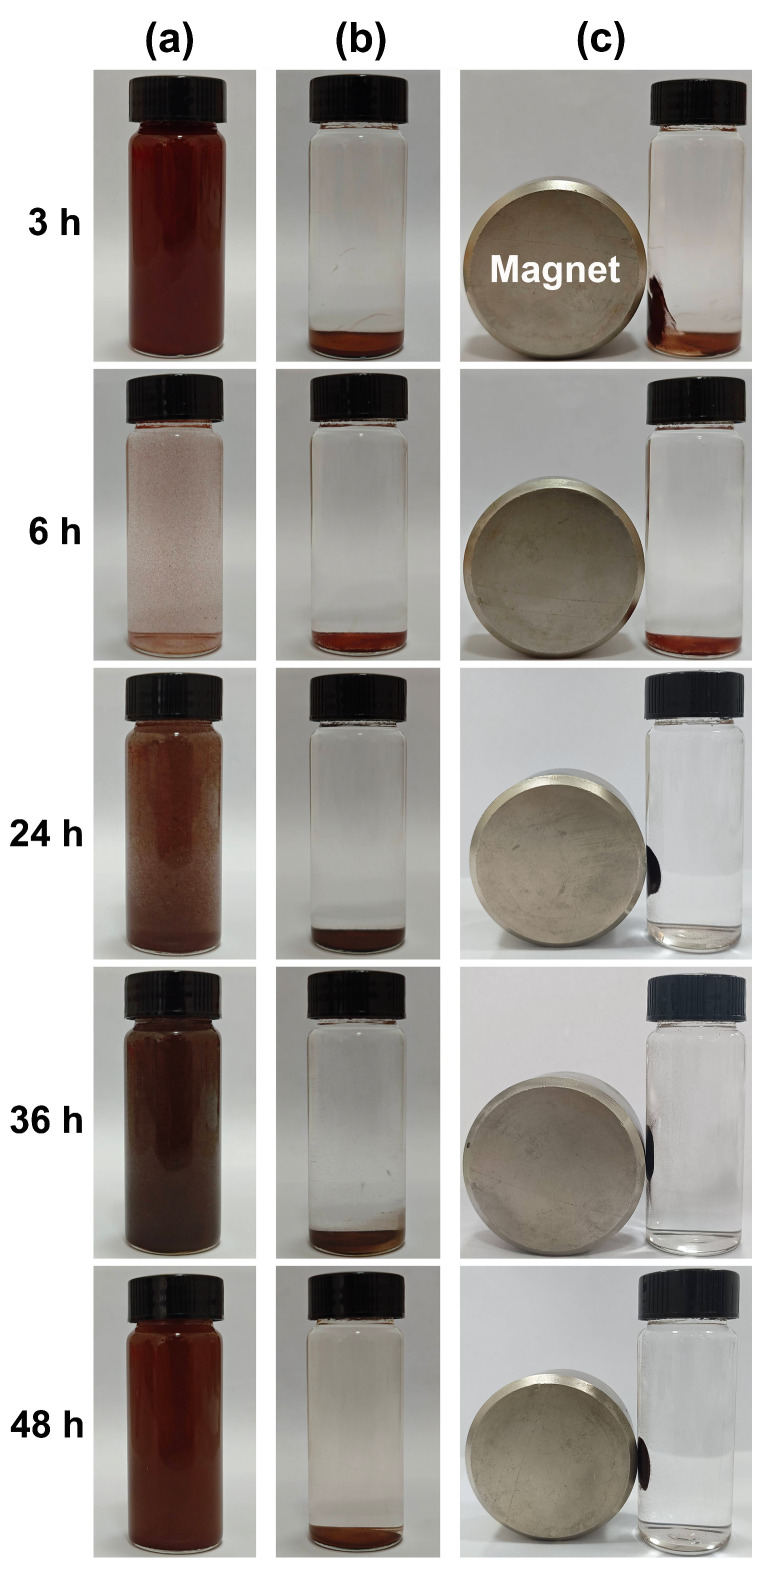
Magnetic response behaviors of the samples synthesized in an organic solvent system using EA at 200 °C for varying durations (3, 6, 24, 36, and 48 h) with a urea amount of 80 mmol: (a) A powder sample weighing 0.1 g was dispersed in a solution of 30 mL H_2_O using ultrasound for a duration of 5 min. (b) The mixture was then allowed to stand for 6 h. (c) Finally, a Nd−Fe−B magnet was placed on one side of the vial for 10 s.
